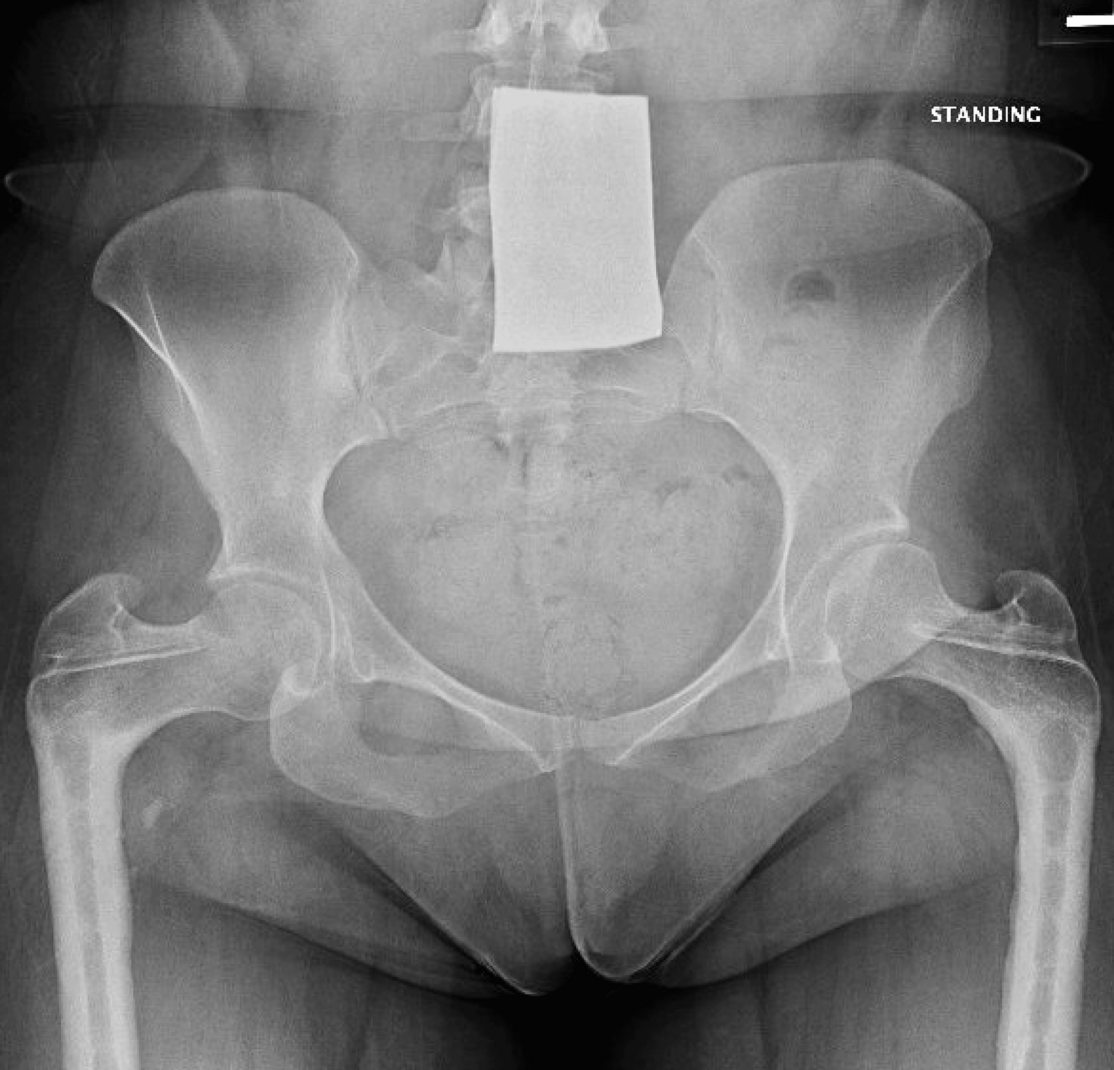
Pre-fracture X-ray showing the neck angle and deformities from the removed hardware
